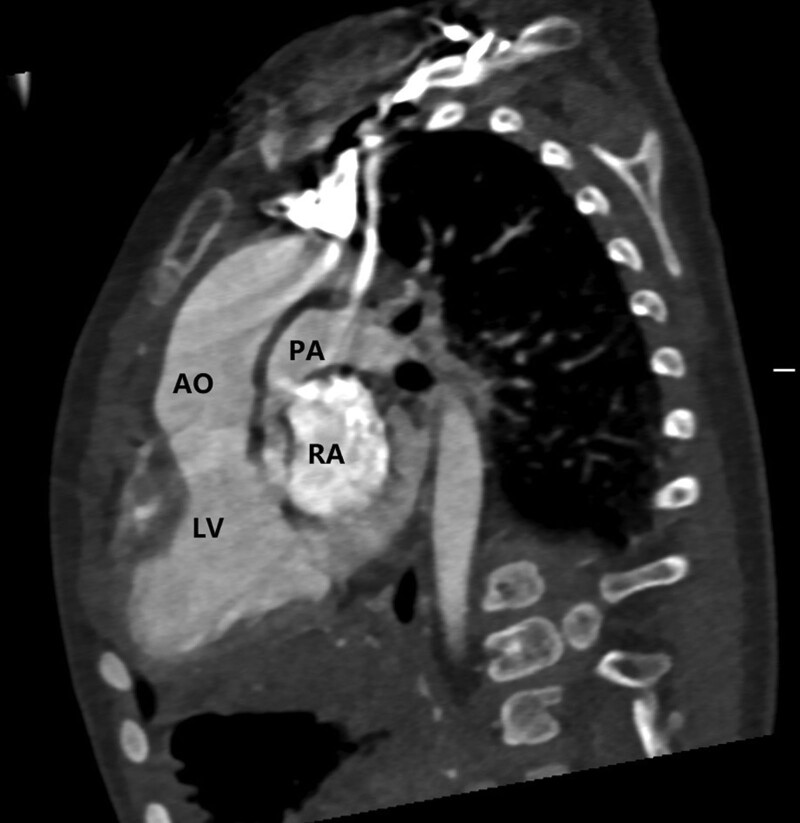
Computed tomography angiography (CTA) shows that the aorta located anterior to the pulmonary artery which was stenosis.
